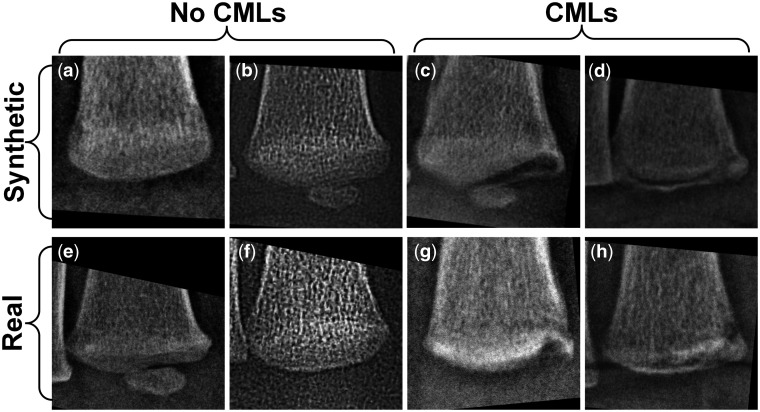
Examples of synthetic (A, B, C, D) and real (E, F, G, H) distal tibial radiographic images, with classic metaphyseal lesions (CMLs) (C, D, G, H) and without CMLs (A, B, E, F). The interpretations of these eight distal tibial images by the three radiologists are presented in Supplemental Material S3.
